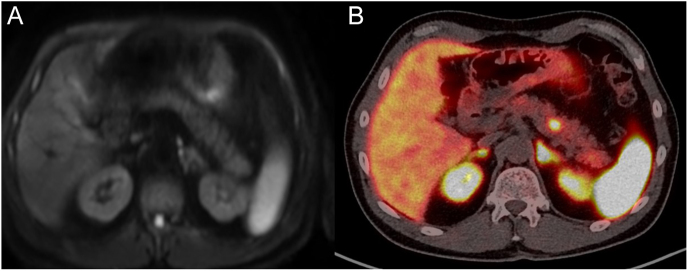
(A) MRI abdomen: difficult to identify pancreatic lesion on MRI. (B) ^68^Ga-DOTA-TATE PET/CT avid pancreatic body lesion.
